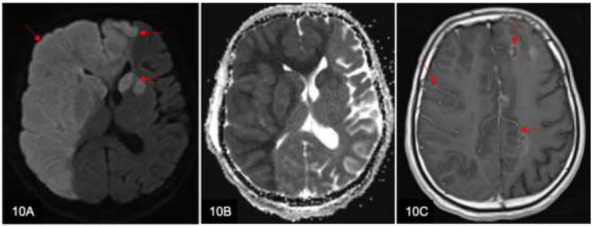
A 57-year-old man presented with seizures. Axial diffusion-weighted imaging (DWI) (A) and axial apparent diffusion coefficient (ADC) (B) MRI brain images show extensive right middle cerebral artery and bilateral anterior cerebral arteries territorial infarcts (arrows) due to occlusion of the right internal carotid artery. Axial T1w post-contrast image (C) shows congested leptomeningeal vessels (arrows) masquerading as leptomeningeal enhancement.
